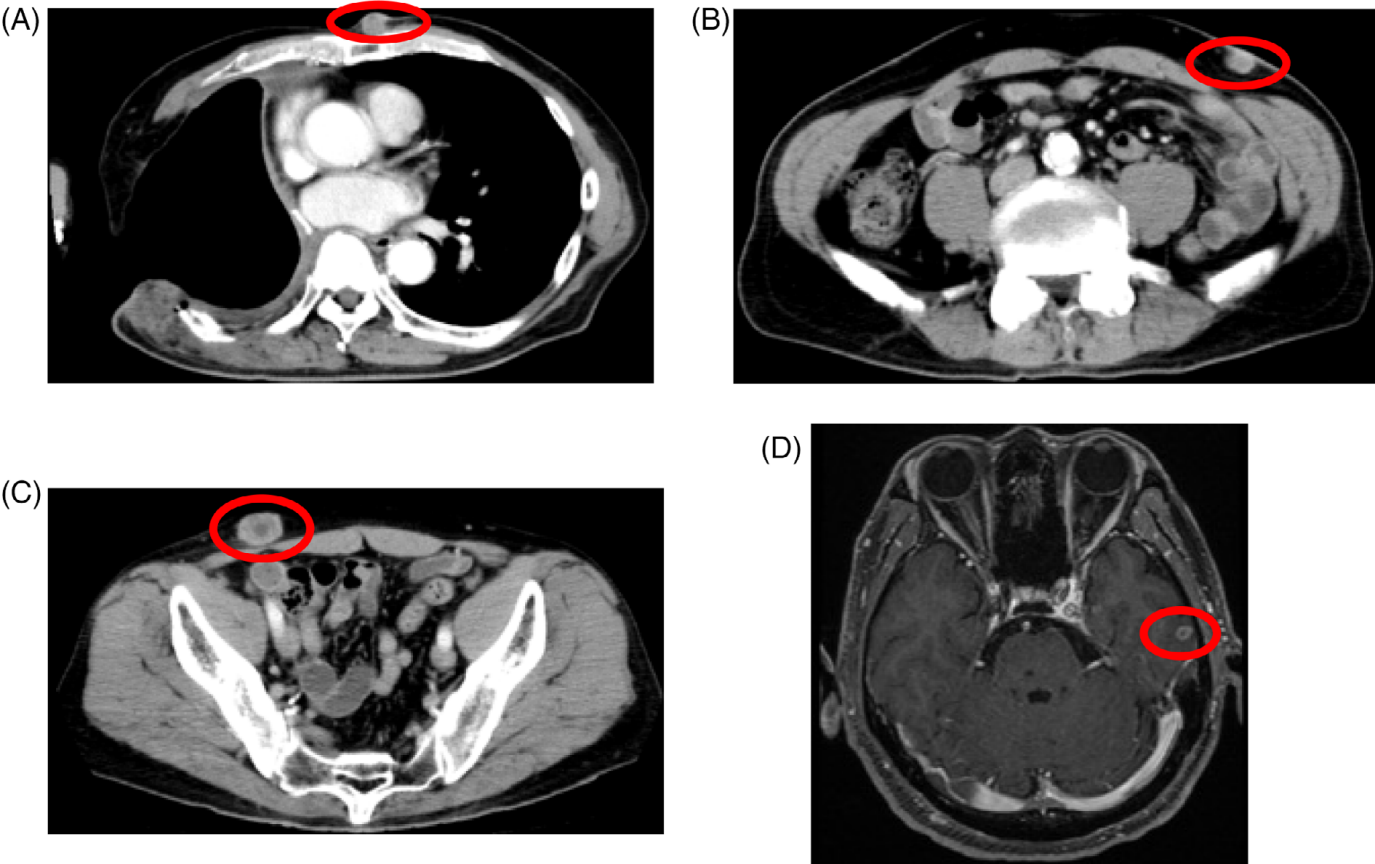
(A–C) Contrast‐enhanced CT and (D) MRI at the time of postoperative recurrence. Thoracoplasty had been performed due to postoperative empyema. A subcutaneous mass in the precordium and multiple subcutaneous metastases were observed on chest and abdominal CT. MRI showed brain metastasis
